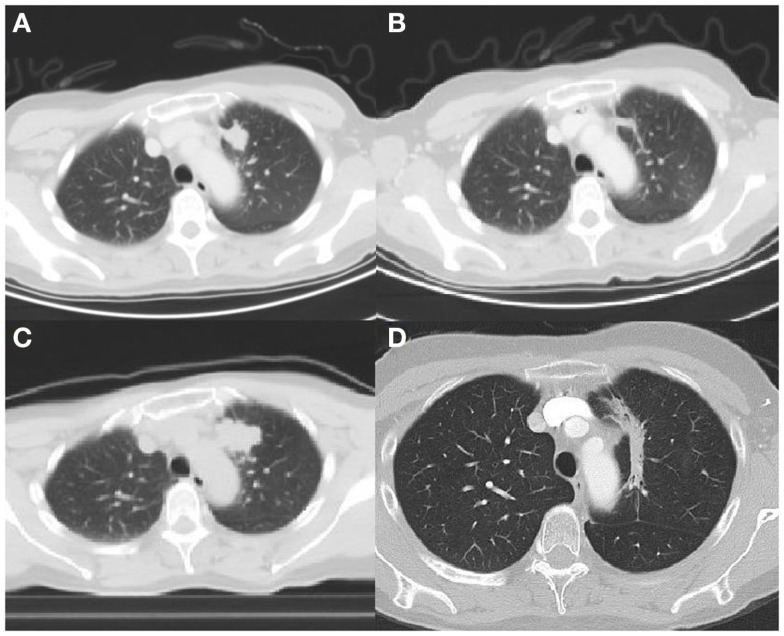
(A) Tumor left upper lobe on diagnosis (18F-FDG PET-CT scan). (B) Near complete response 32 months after start TKI (18F-FDG PET-CT scan). (C) Local progression 40 months after diagnosis (18F-FDG PET-CT scan). (D) Partial response 8 months after local radiotherapy (CT scan).
